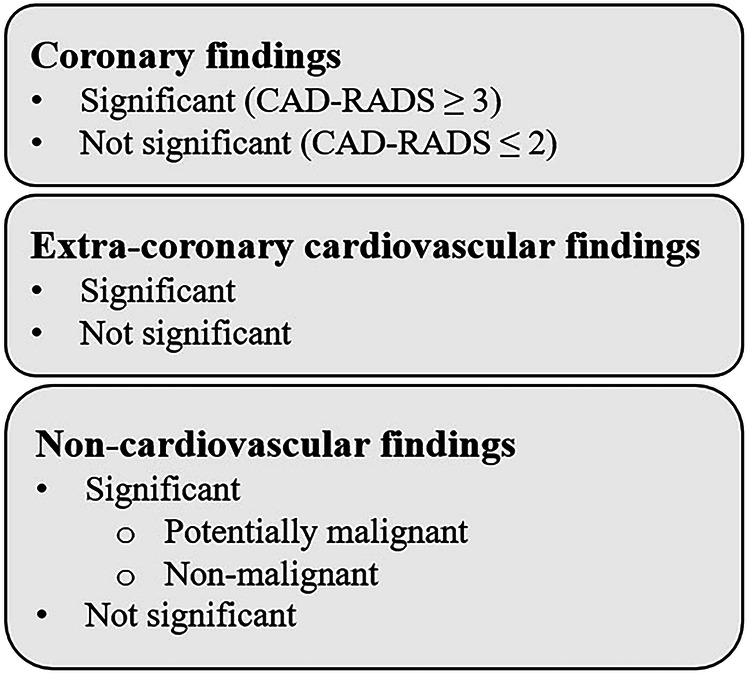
Classification scheme of findings on coronary computed tomography angiography (CCTA). Findings were categorized into coronary, extra-coronary cardiovascular, and non-cardiovascular findings, and stratified by clinical relevance. Non-cardiovascular findings were further subdivided into potentially malignant and non-malignant categories. Data were analyzed and reported at the patient level; patients could contribute to more than one subcategory
